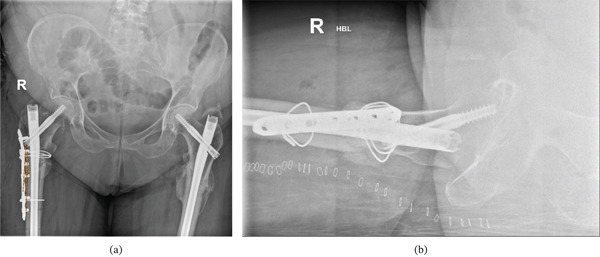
(a) Day 2 postoperative anteroposterior radiograph showing the satisfactory valgus neck‐shaft angle after revision fixation of right femur with long cephalomedullary nail and valgus correction. Noted valgus alignment postrevision fixation (measured 2°) compared with initial varus angle measured 14°(Figure 3) and (b) Day 2 postoperative lateral radiograph of right femur following revision fixation with long cephalomedullary nail and valgus correction.
